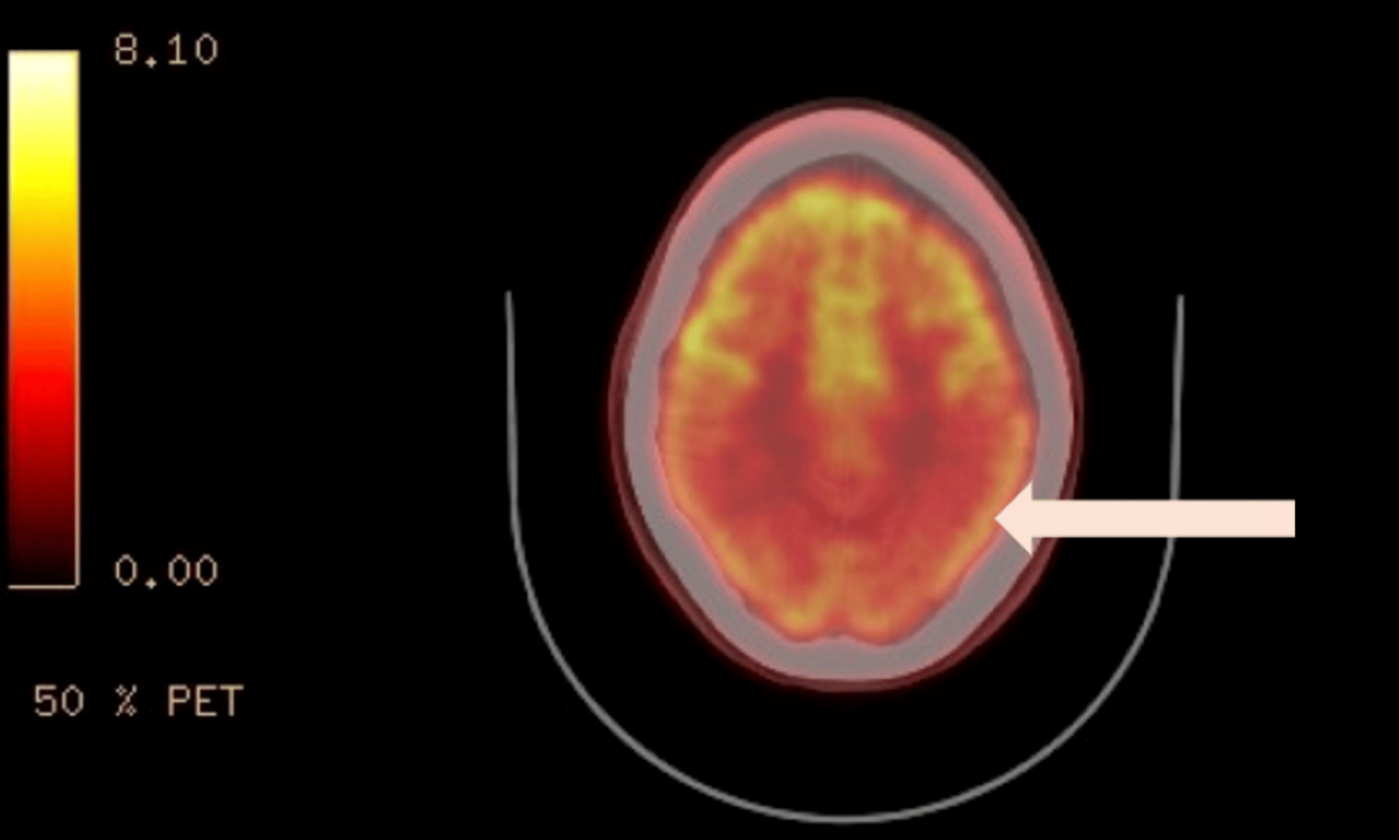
Axial fused 18F-fluorodeoxyglucose positron emission tomography/computed tomography (18F-FDG PET/CT) demonstrates moderate-significant decreased FDG uptake in the bilateral parietal lobes. The white arrow points to bilateral parietal lobes with FDG hypometabolism, signifying neuronal dysfunction and potential neurodegenerative changes.
